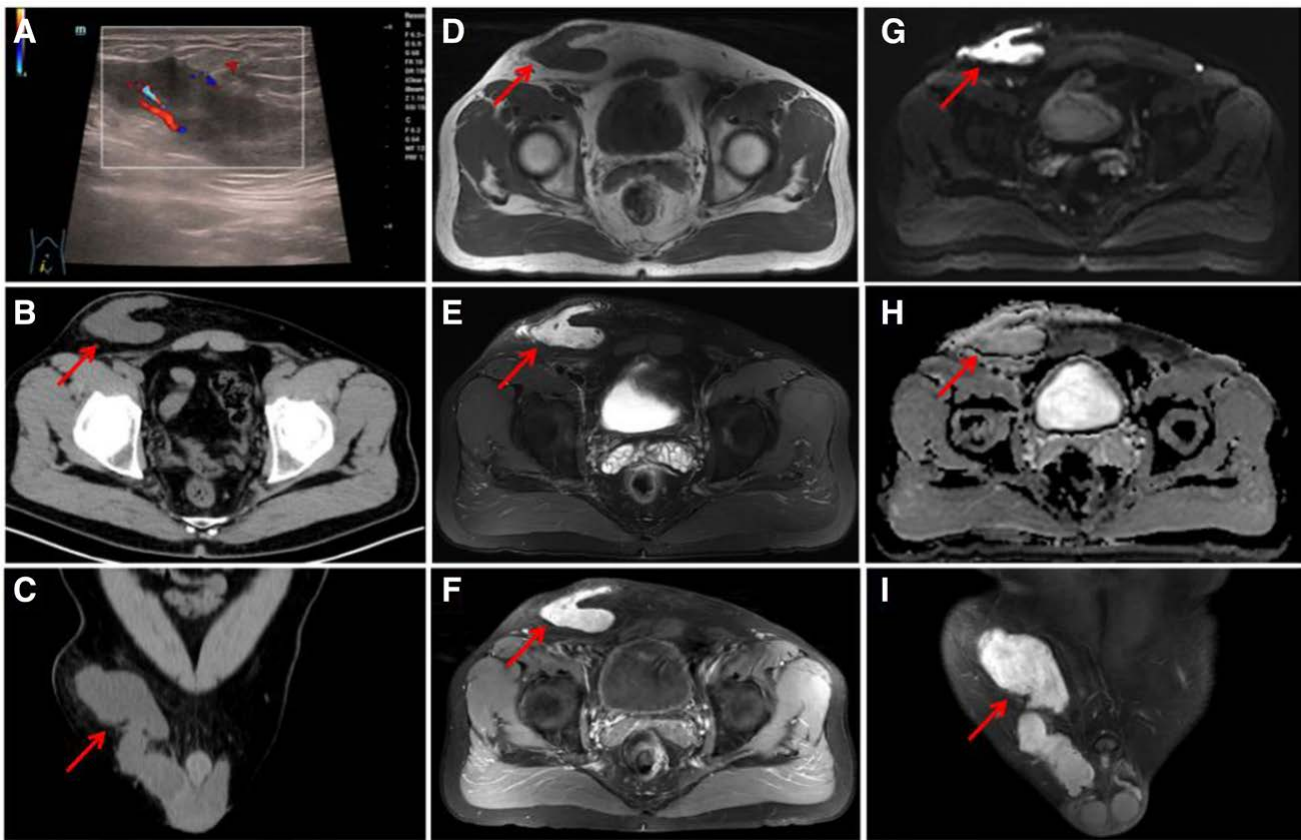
Patient scans. The lesion is indicated by the red arrow. (A) Ultrasonography showed a hyperechoic mass (15.0 × 6.1cm) in the right inguinal region with irregular morphology, with clear borders, and a small amount of blood flow signal could be seen within it. (B and C) Pelvic CT showed a soft tissue density mass (15.3 × 6.1cm) in the right inguinal region with irregular morphology and clear borders. (D–I) Pelvic MRI showed an irregular isotropic T1 long/slightly long T2 signal soft tissue mass (15.5 × 6.0cm) shadow in the right inguinal region, part of the level seemed to be connected with the right peritesticular adnexa, enhancement was visible, DWI was high signal, and ADC signal was not low. ADC = apparent diffusion coefficient, CT = computed tomography, MRI = magnetic resonance imaging.
